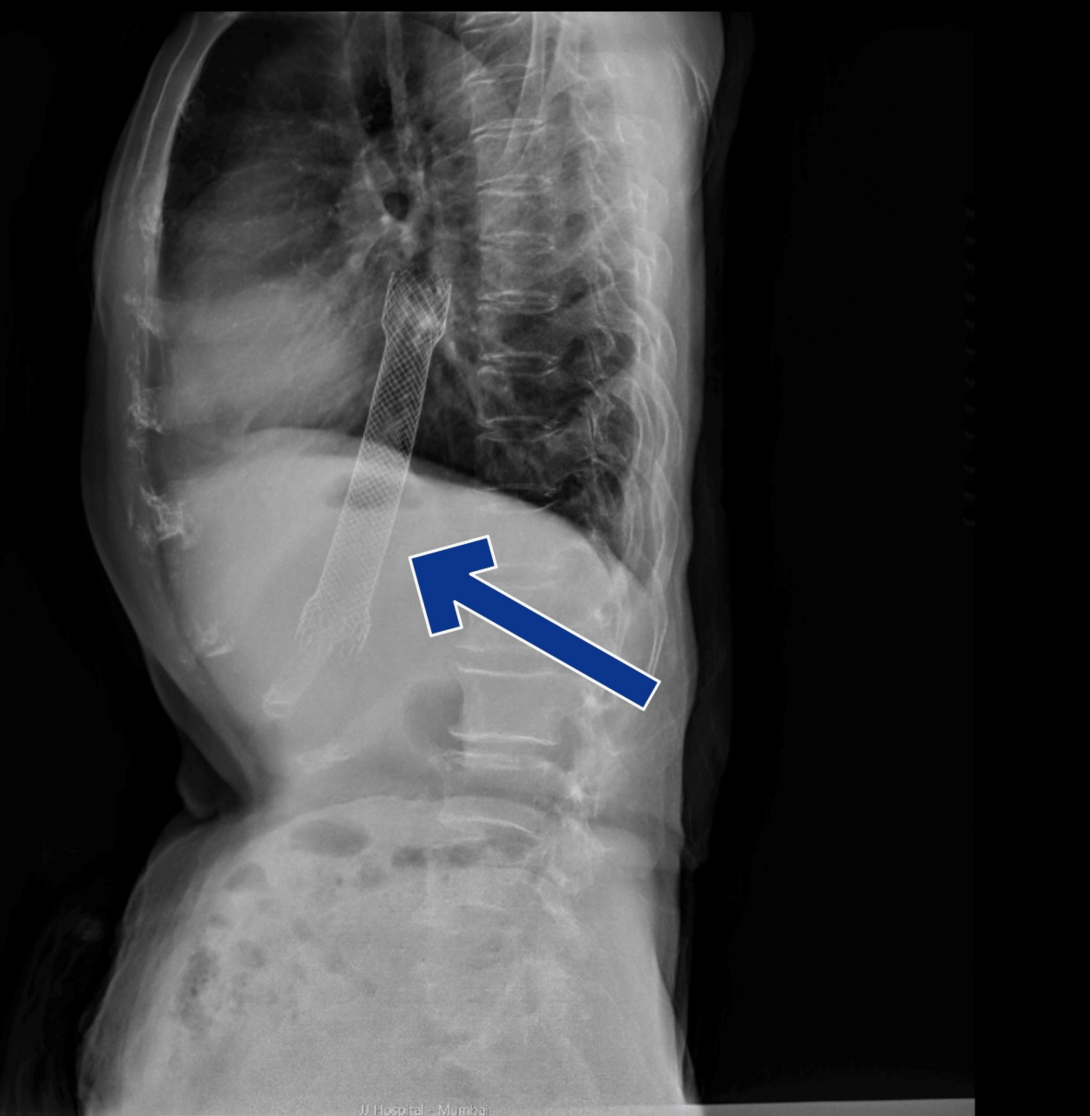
X-ray lateral view of thorax showing the distally migrated metallic stent (blue arrow)
